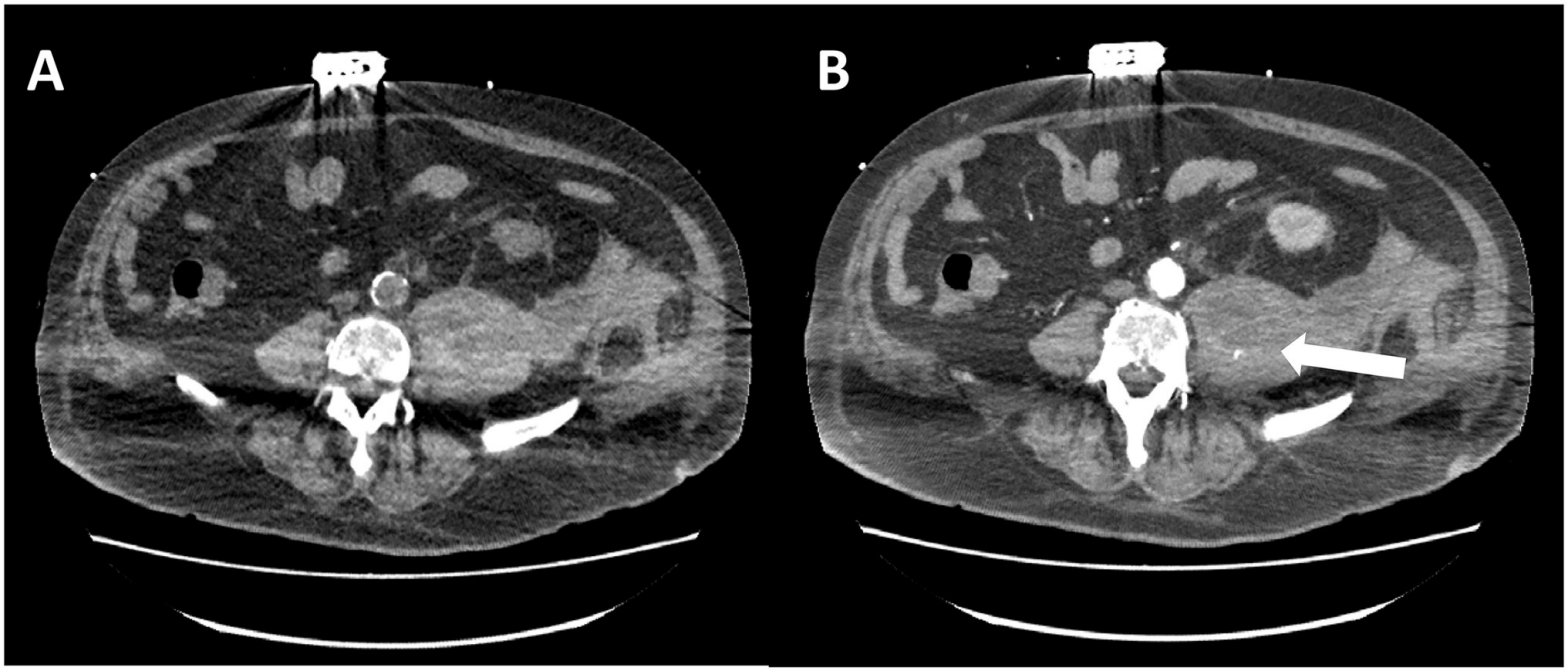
Spontaneous left psoas hematoma in a 70-year-old patient. (A) Axial CT scan of the abdomen without contrast shows swelling of the left psoas with retroperitoneal diffusion (B) Axial contrast-enhanced CT scan shows extravasation of contrast medium (arrow).
